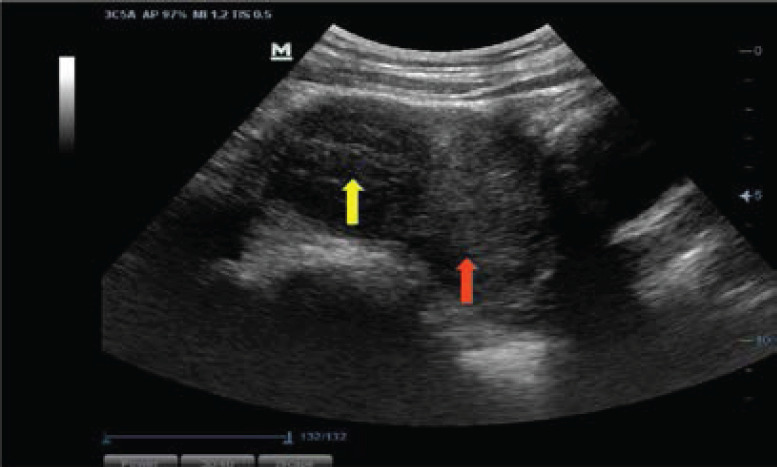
A longitudinal ultrasound image of the uterus showing a centrally fundal myoma (yellow arrow) and a posterior lower uterine myoma (red arrow)
